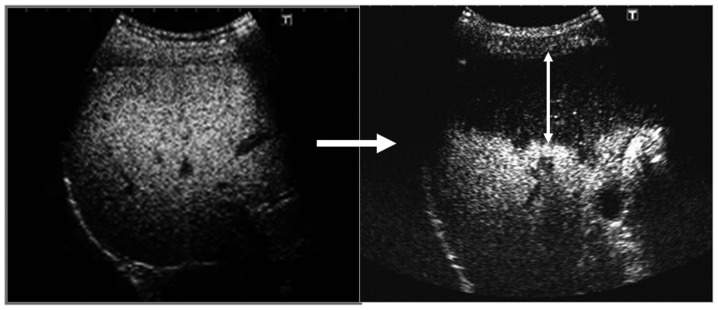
Measurement of microbubble collapse in the post-vascular phase of Sonazoid-enhanced ultrasonography 10 min after contrast infusion. The focus point was 6 cm. Flash-replenishment sequence settings included a mechanical index of 1.6 and number of beam transmissions set at 30. Then, we measured the depth of microbubble collapse from the liver surface after destroying the microbubbles in the scan volume with the use of high-transmission power ultrasound.
